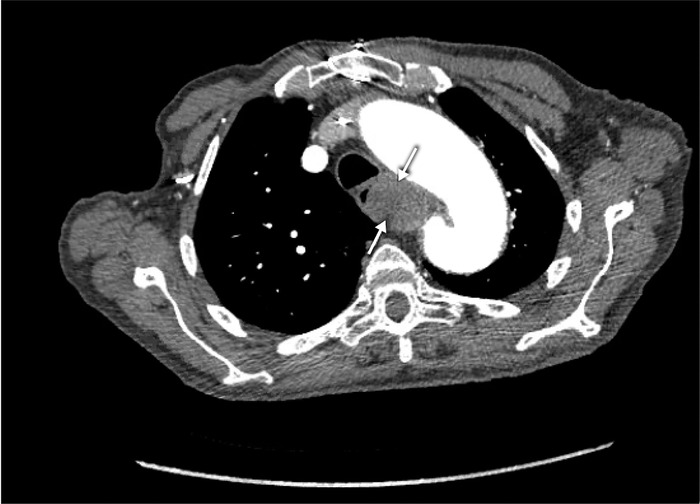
Computed tomography angiography of the chest demonstrates an ascending thoracic aortic aneurysm with saccular pseudoaneurysm formation. Arrows point to the aneurysm compressing the esophagus.
